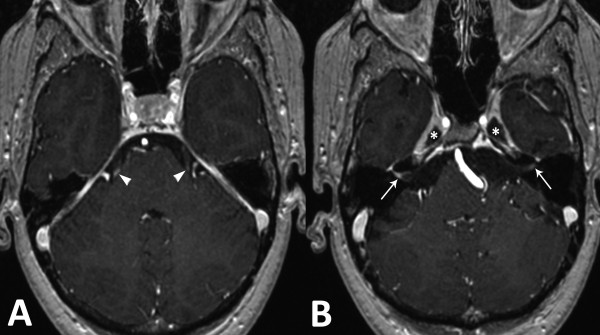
Axial MRI images of the brain. Post gadolinium enhanced T1 weighted sequence on initial exam shows (A) bilateral focal enhancement of the proximal portion of both trijeminal nerves (arrowheads), (B) bilateral enhancement of the vestibulocochlear and facial nerves, more pronounced on the right side (arrow) and bilateral thickening of the Gasser’s ganglia walls (*).
